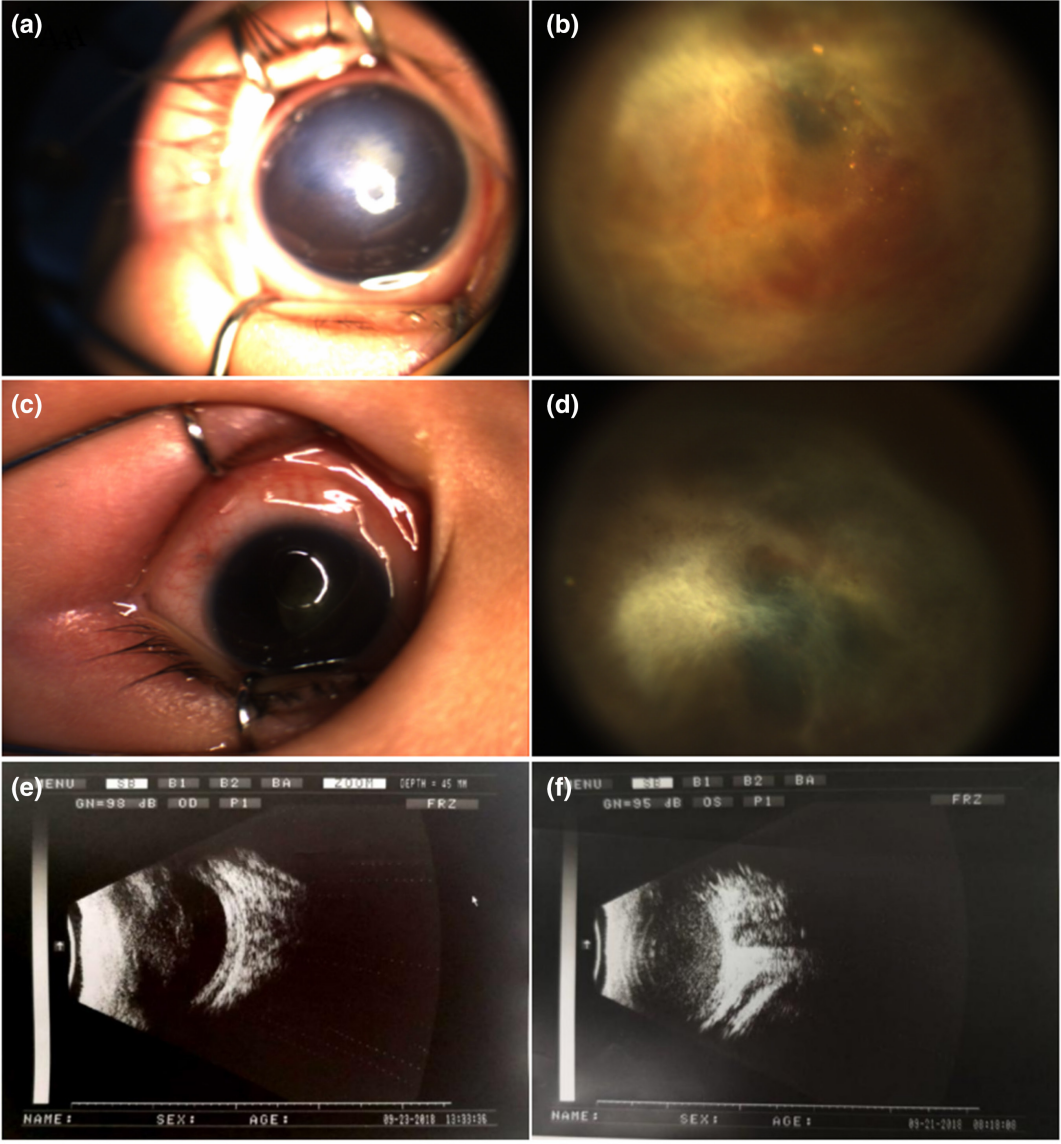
Photographs of the anterior segment and B‐mode ultrasonography findings of case IV‐2: (a, b, e) represent the right eye, (c, d, f) represent the left eye. (a, b) The photographs of right eye showed no light sensation, a white spot about 3 × 3 mm, the anterior chamber disappeared, the pupil was not round, part of the iris was attached to the inner skin of the cornea, the lens was mildly cloudy, a large amount of golden granules were hidden in the vitreous cavity, and retinal detachment was hidden in the fundus. (c, d) The left eye showed no light sensation, the cornea was clear, the anterior chamber was deep, the pupil was not round, the crystal was cloudy, retinal hemorrhage was hidden in the fundus, and total retinal detachment was observed. (e) The morphology of right eyeballs was fine, and diffuse spot cluster echo was visible in the vitreous cavity. (f) Diffuse mass echoes are seen in the vitreous cavity of the left eye.
